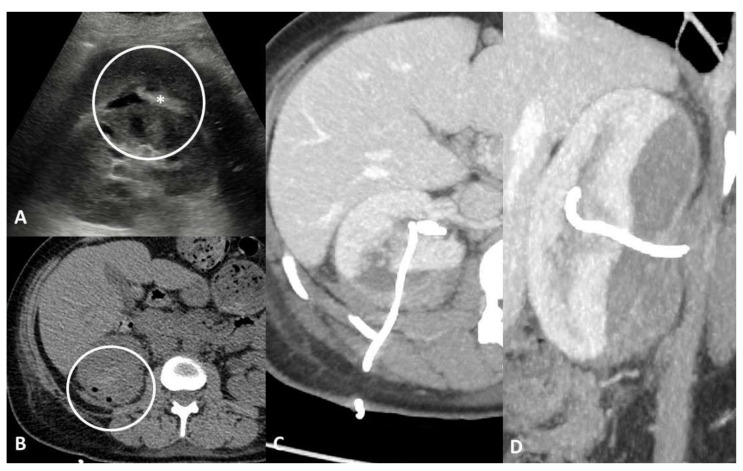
Parenchymal/tissue injury. Forty-one-year-old female with persistent haematuria after percutaneous nephrostomy placement. Axial ultrasound scan (A) shows hypoechoic collection of the right kidney with hyperechoic material inside (*). Non-enhanced CT scans (B) confirm the presence of a subcapsular hematic collection associated with a heterogeneous density area in the posterior renal parenchyma. Axial (C) and coronal (D) maximum intensity projection CT images show more clearly the large parenchymal laceration and the direct relationship with the nephrostomy tube, without signs of active bleeding.
